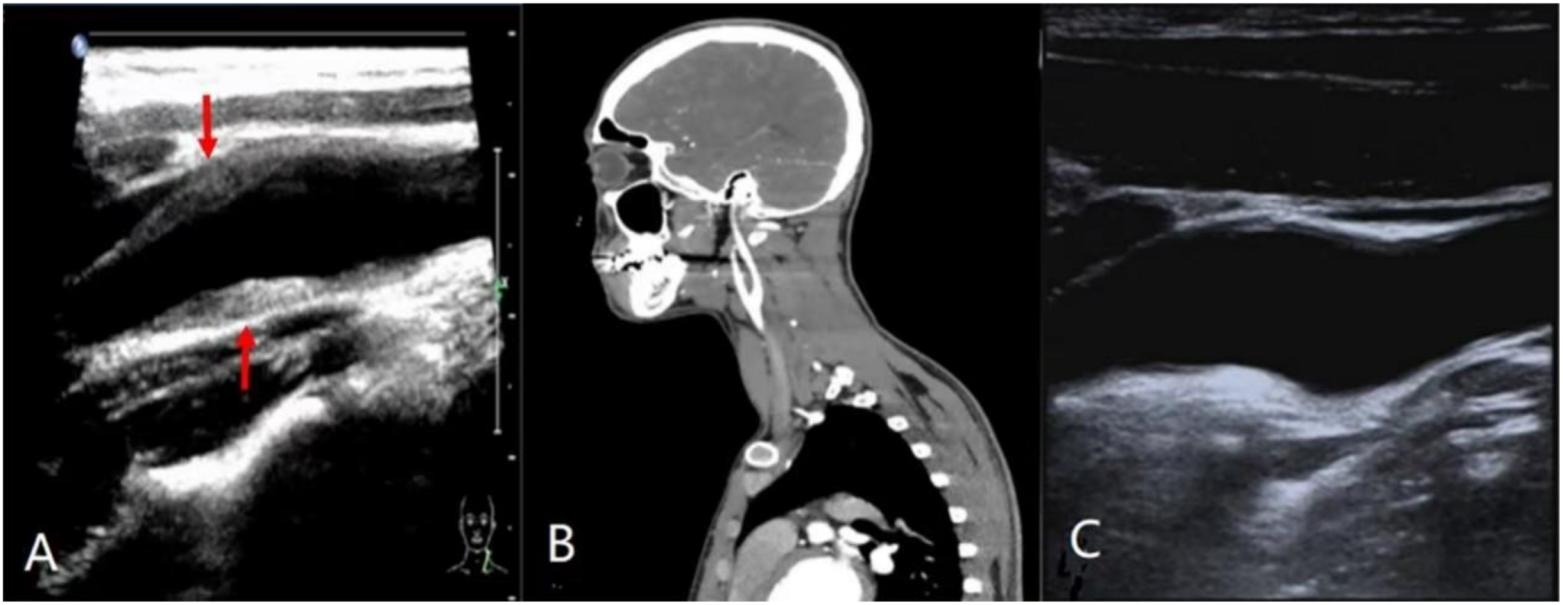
Imaging presentation of a patient with TIPIC syndrome: (A) Diagnostic ultrasound shows a bifurcation of the carotid artery with a deviated perivascular infiltrate (arrow), the presence of a mild luminal stenosis, no hemodynamic changes. (B) Patient underwent Computed Tomographic Angiography a few days after treatment, suggesting complete normalcy. (C) Ultrasound follow-up after one month shows disappearance of eccentric infiltrates in the carotid bifurcation, with lumen patency restored.
